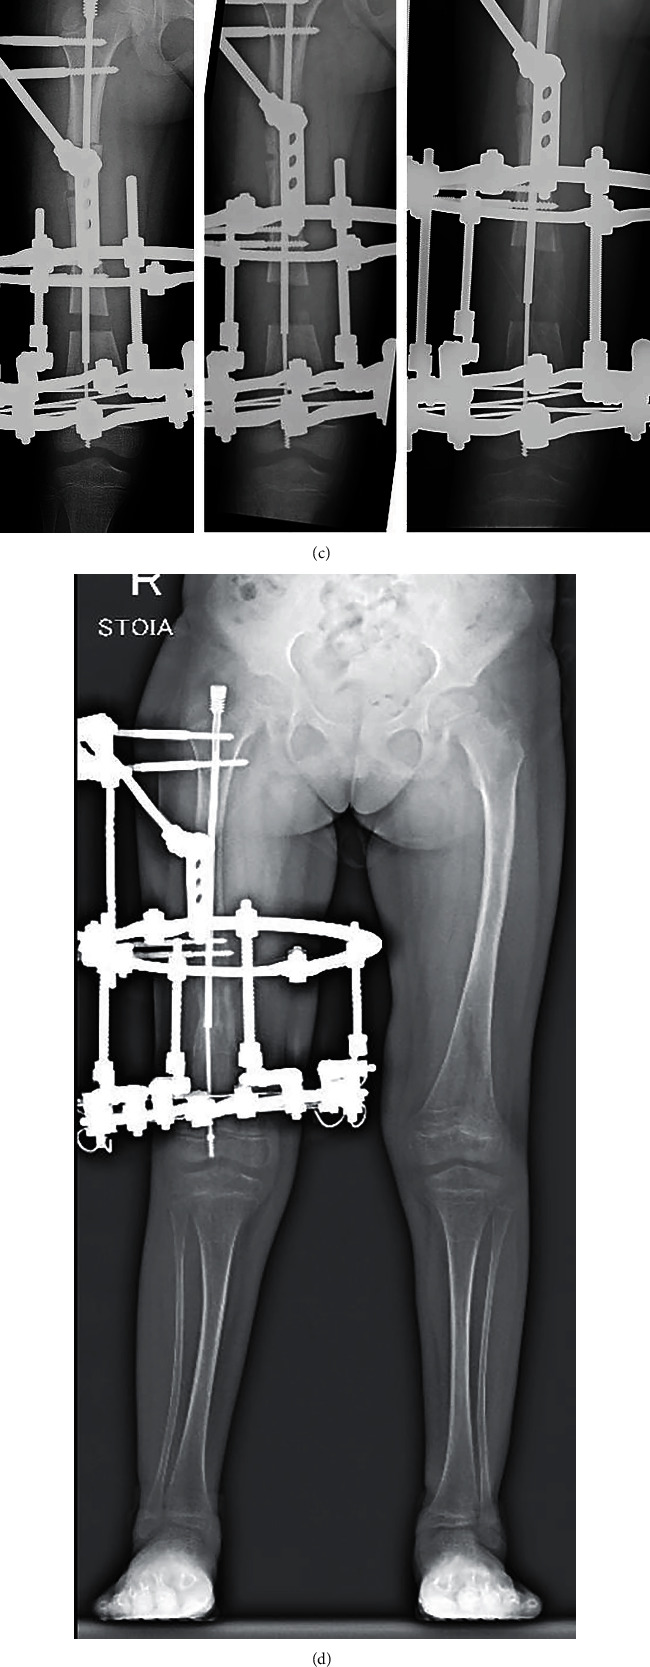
Patient S., osteogenesis imperfecta, type I, radiographs: (a) preoperative full-size standing radiographs; (b) intraoperative radiographs: double osteotomy of the right femur. Note open-wedge proximal osteotomy; (c) consecutive radiographs demonstrating increasing gap between fragments and developing bone regenerate during distraction period; (d) full-size standing radiograph of lower limbs, satisfactory alignment and lengthening of right limb; and (e) full-size standing radiograph of lower limbs in 3 months after frame removal, satisfactory alignment of the lengthened of right limb (anatomical position of the mechanical lower limb axis).
